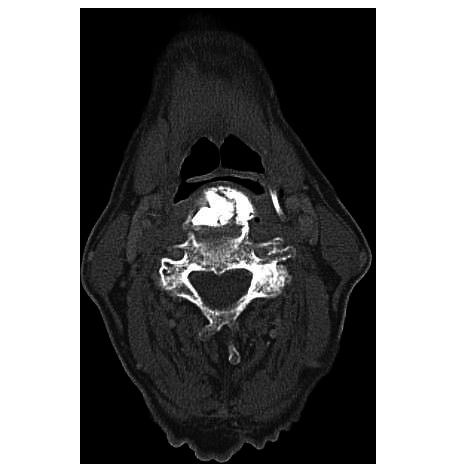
CT: axial scans showing a thin cleft between epiglottis and anterior osteophytes at the level of C3.
